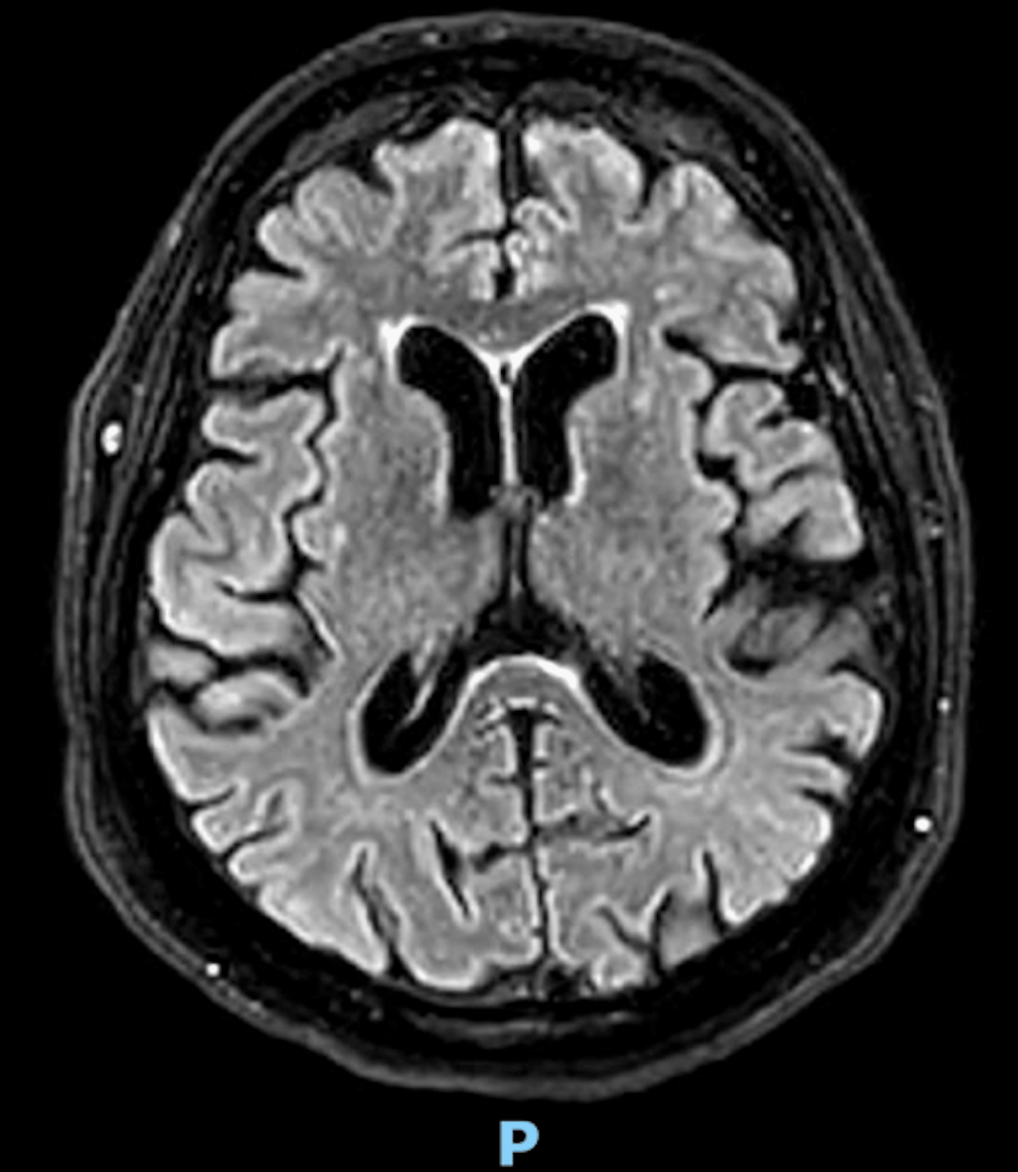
November 2021: MRI head showing mild small vessel disease, but no significant reduction in the size of the brain or any intracranial abnormalities
